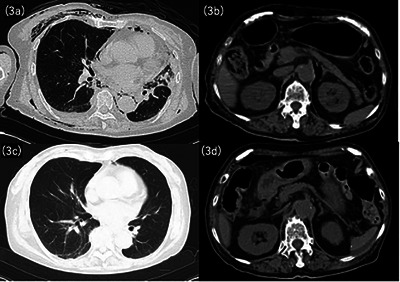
Computed tomography (CT) findings immediately after intraoperative perforation and at the time of postoperative duodenal ulcer perforation. (a) Chest CT immediately after intraoperative perforation, showing subcutaneous and mediastinal emphysema. (b) Abdominal CT immediately after intraoperative perforation, showing retroperitoneal emphysema due to endoscopic submucosal dissection (ESD) perforation without intra‐abdominal free air. (c) Chest CT 6 days postoperatively, demonstrating resolution of mediastinal and subcutaneous emphysema. (d) Abdominal CT 6 days postoperatively, showing the disappearance of retroperitoneal emphysema, but revealing intra‐abdominal free air and fluid collection at the posterior aspect of the duodenal bulb.
